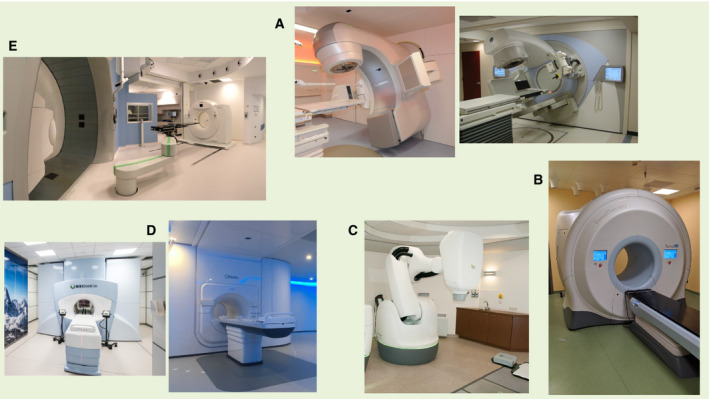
Examples of available ‘High‐Tech image‐guided Linacs’: (A) Conventional Linacs equipped with cone‐beam CT [Zurich UH (left) and NKI‐AvL, Amsterdam (right)]: The kV imaging system is perpendicular to the beam axis and CT images are obtained by rotating the gantry; (B) Helical delivery system (OSR, Milano): The Linac is integrated into a CT ring: Megavoltage images are obtained by using the same treatment fan beam paired to a detector array, delivered in a helicoidal way by moving the couch; (C) Robotic system (Erasmus, Rotterdam): Dose delivery is generated by a large number of small noncoplanar beams delivered by the robotic arms, while image guidance (including tracking during delivery) is driven by a perpendicular pair of flat panel on the floor (in the right corner of the picture) paired to two kV X‐rays tubes positioned on the room ceiling; (D) Hybrid MRI‐Linac machines [Zurich UH (left) and NKI‐AvL, Amsterdam (right)]: The Linac is integrated into an MRI. MRI images can be obtained before and during the treatment delivery. (E) Proton system (Protontherapy, Trento): The gantry (left of the picture) is paired to a robotic couch and a diagnostic CT in the room to setup the patient before treatment delivery
